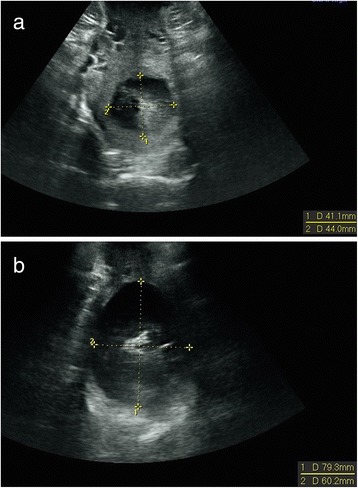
Transperineal (2D) ultrasound measurement of cervical dilatation at (a) 4,3 cm and (b) 7,0 cm
